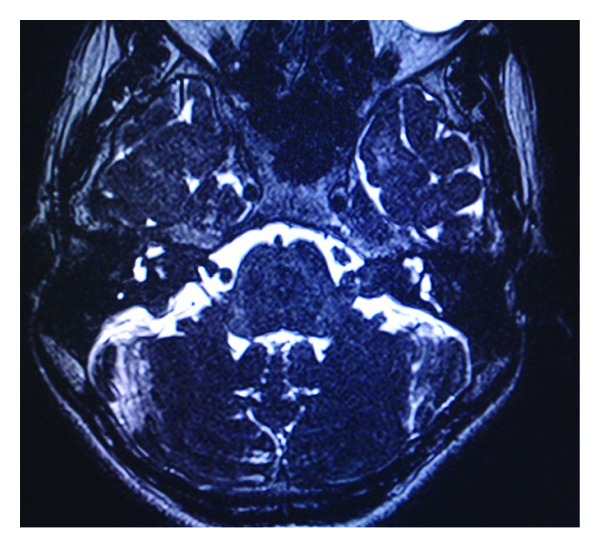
Axial constructive interferance in steady state MR image showing bilateral acoustic schwannomas with internal auditory meatus widening and CP angle cisternal extension.
